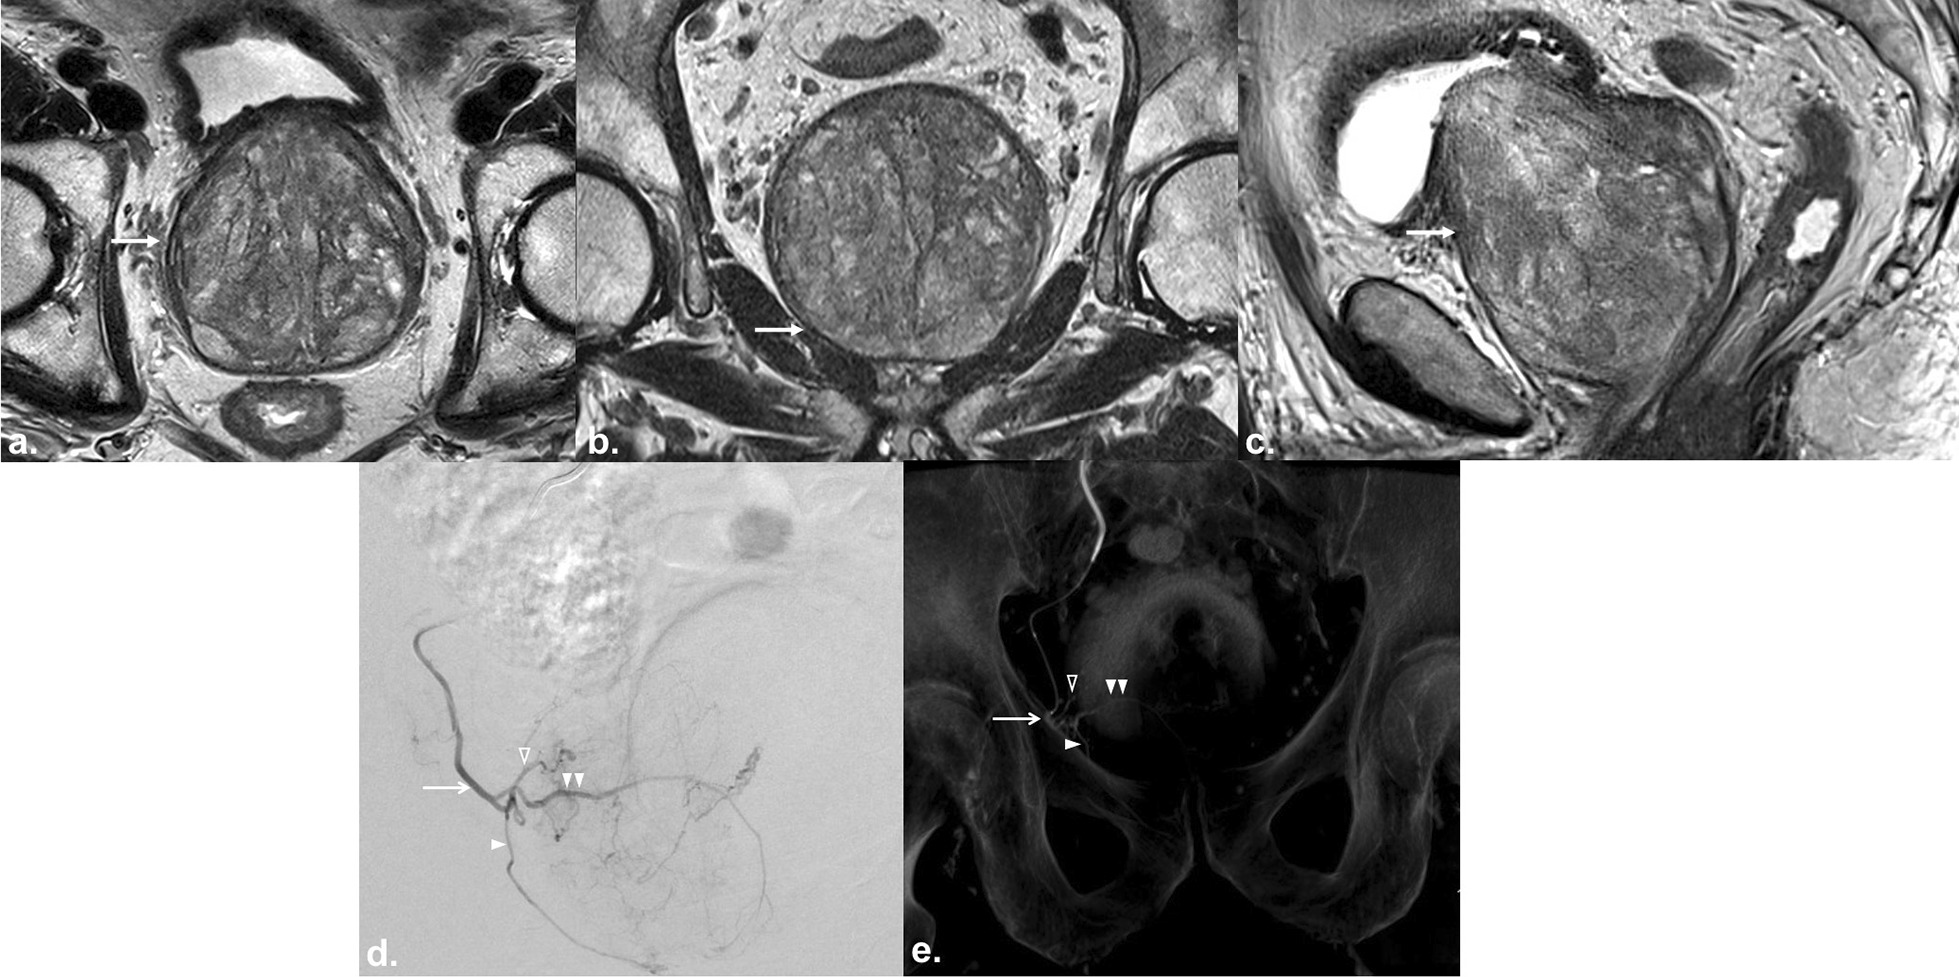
A 71 year-old patient with severe lower urinary tract symptoms (LUTS) from giant prostatic hyperplasia (GPH) underwent prostate artery embolization (PAE). a Axial T2-weighted turbo spin echo (TSE) image, b coronal T2-weighted TSE image, and c sagittal T2-weighted image from multiparametric magnetic resonance (MR) imaging show giant hyperplasia of the prostate gland (arrow) that measures 312 mL in volume. d Digital subtraction angiography (DSA) of selective catheterization of the right internal iliac artery anterior division shows a common origin of the right prostatic artery (straight arrow), which is hypertrophied, and the right superior vesical artery (open arrowhead). The anterior/lateral prostatic artery (single arrowhead) and the posterior/lateral prostatic artery (double arrowheads) are hypertrophied with a corkscrew pattern of the intraprostatic arterioles. e Cone-beam computed tomography (CT) with intravenous contrast in the coronal plane after selective catheterization of the internal iliac artery anterior division shows the anatomy of the right prostatic artery: a common origin of the right prostatic artery (straight arrow) and the right superior vesical artery (open arrowhead), hypertrophy of the anterior/lateral prostatic artery (single arrowhead) and the posterior/lateral prostatic artery (double arrowheads), and no vascular supply to the adjacent anatomical structures, to include the urinary bladder, penis, and rectum
